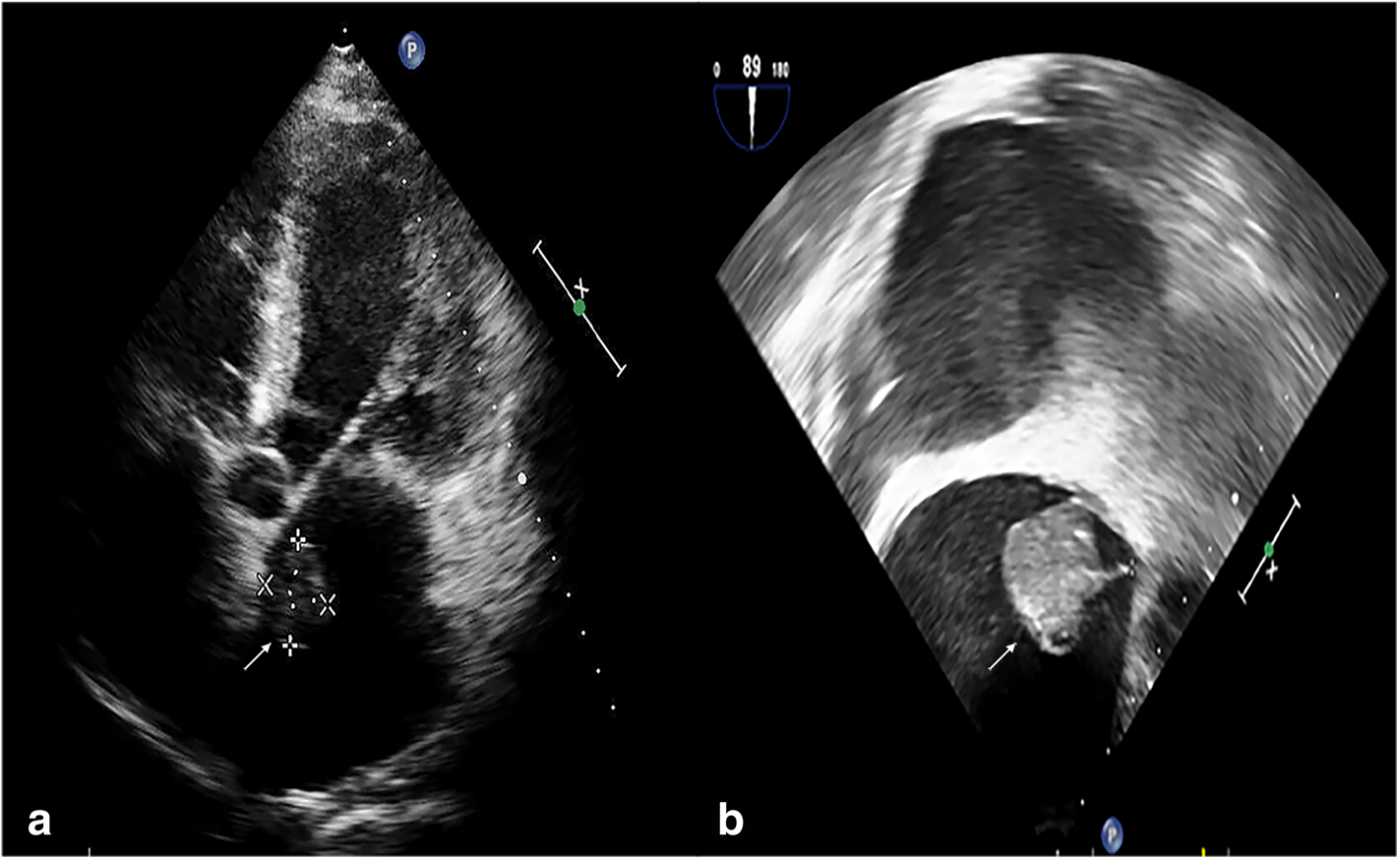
a Transthoracic echocardiography and (b)transesophageal echocardiography showing a 2.7 cm × 2.6 cm hypoechoic mass (arrow) attached by a thin stalk (measuring 0.15 cm in size) to the base of the atrial apex, freely swing within the left atrial cavity
